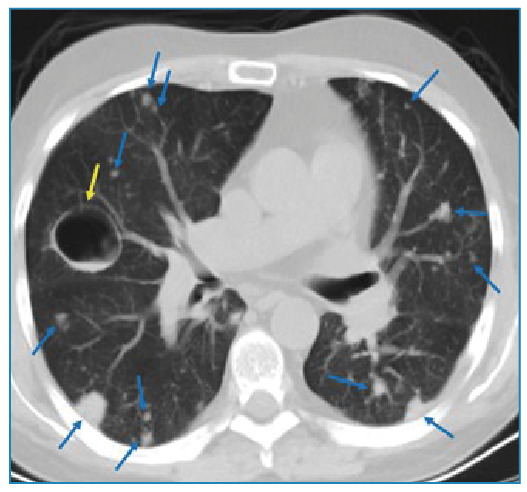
Thoracic CT showing cavitary (yellow arrow) and multiple solid nodules in both lungs (blue arrows).
